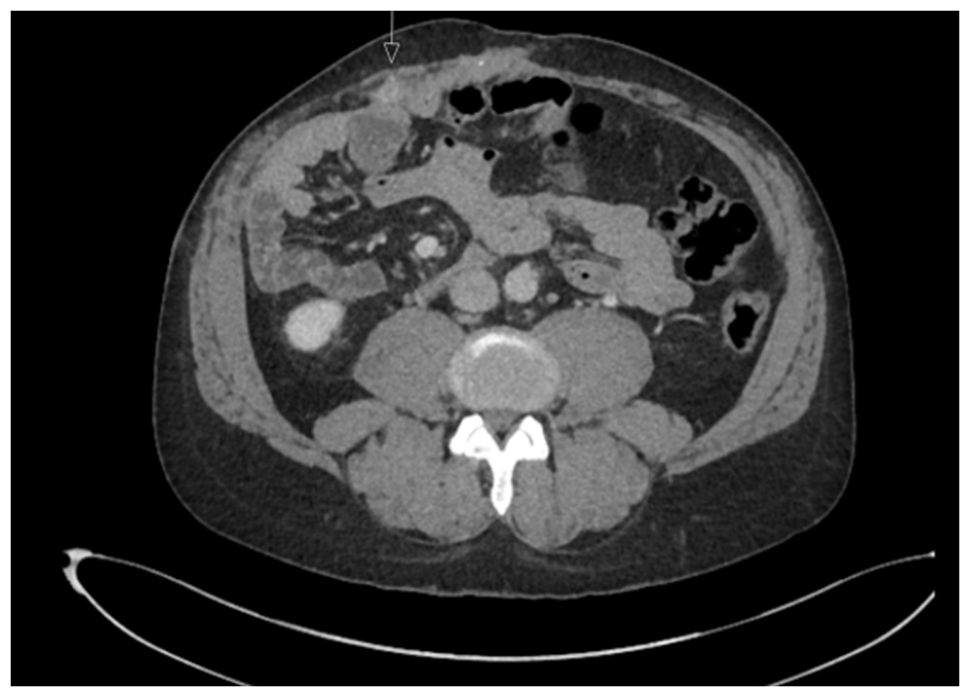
Axial computed tomography demonstrating an FDG-avid soft tissue lesion in the anterior rectus sheath, consistent with metastatic cholangiocarcinoma (white arrow).
